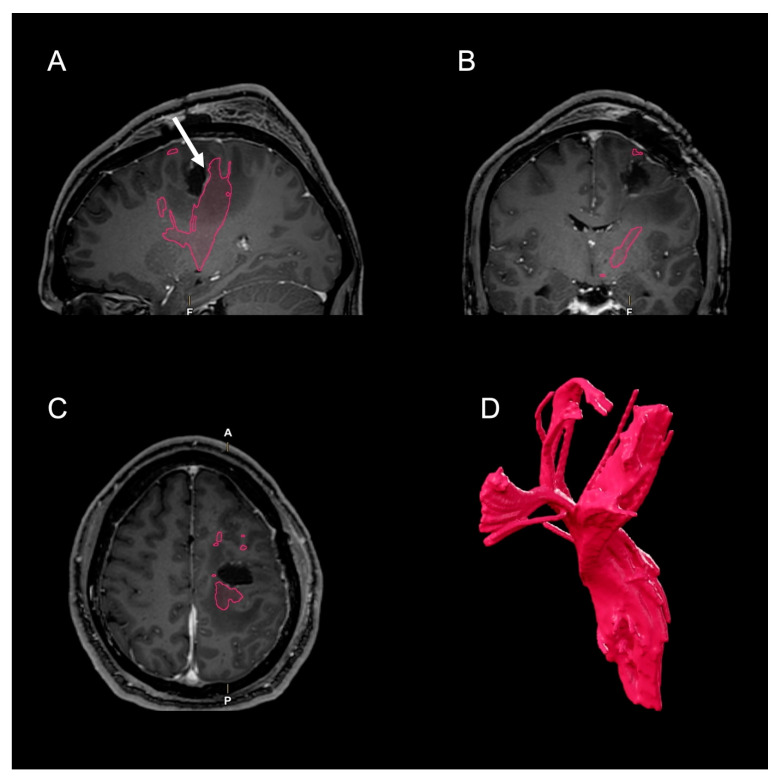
Postoperative MRI in T1-weighted sequence with contrast enhancement (A–C). Arrow: location of positive intraoperative DES at 2 mA. The contrast-enhancing parts of the tumor were completely resected. Edema was already reduced. Red outlines: CST. (D) Postoperative tractogram of the left CST (red) still shows some fibers ventral to the situs. However, the dorsal parts appear much stronger than preoperatively. (MRI: magnetic resonance imaging; CST: corticospinal tract; DES: direct electrical stimulation).
